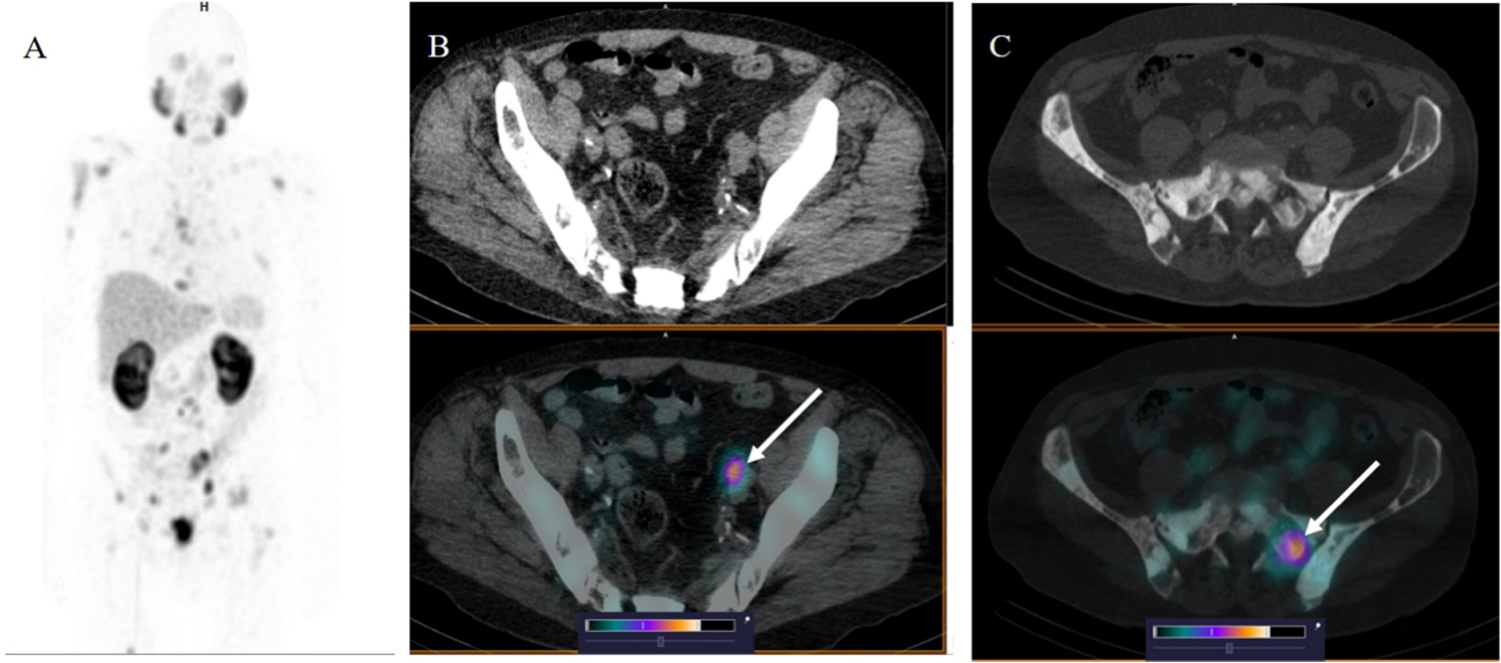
SPECT image 2 h after injection of [^99m^Tc]Tc-BQ0413
